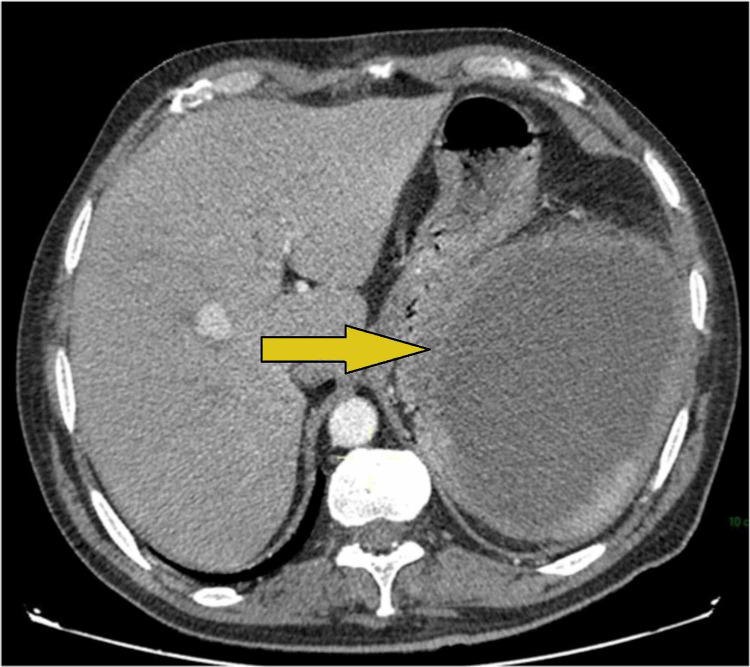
Axial section of abdominal CT scan showing a splenic abscess.
